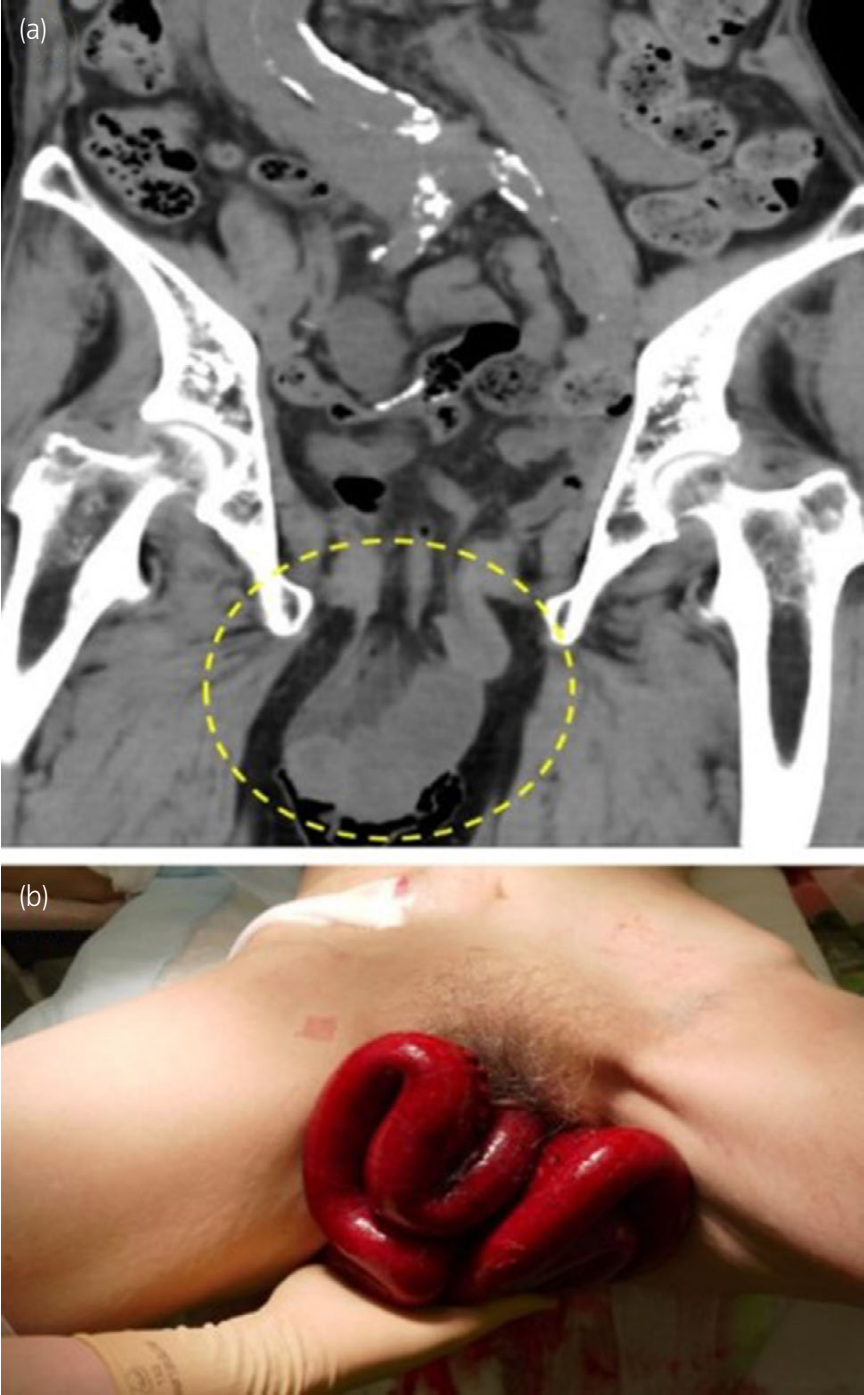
Computed tomography image of the eviscerated small bowel and gross appearance of the necrotic eviscerated small bowel. (a) The small bowel was eviscerated through the vaginal cuff. (b) The color of small bowel was reddish black in color and seemed to be necrotic.
